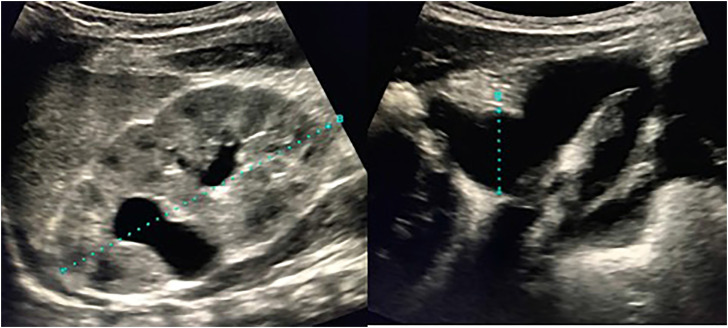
Preoperative ultrasound demonstrating severe hydroureter and hydronephrosis to the upper pole moiety of a duplex collecting system.
